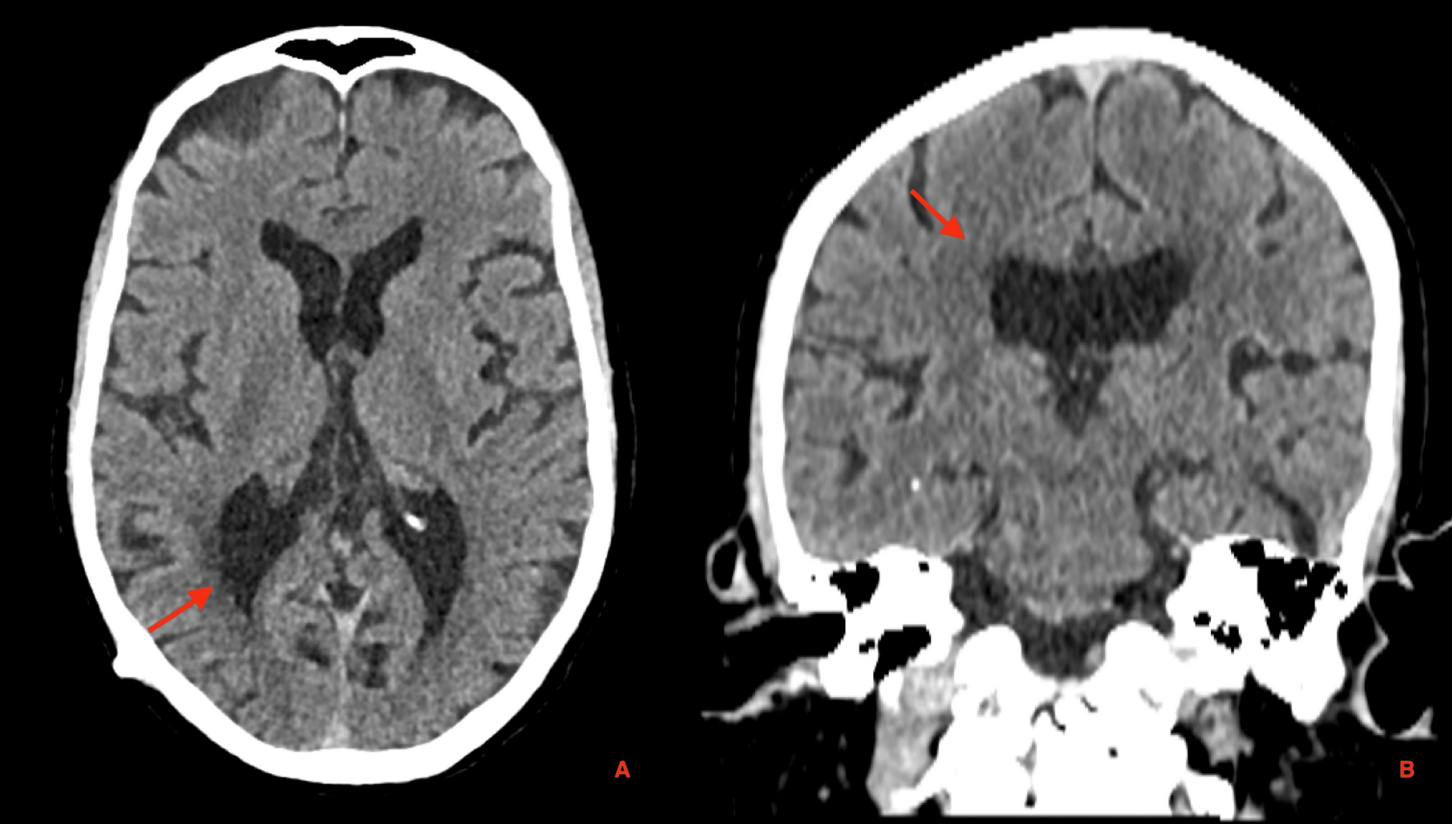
Two-week follow-up cranial CT demonstrating complete resolution of the previously observed intraventricular pneumocephalus and left temporal encephalomalacia. (A) Axial and (B) coronal reconstructions show normal ventricular configuration and absence of intracranial air, confirming full radiological resolution after conservative management (red arrow). CT: computed tomography
